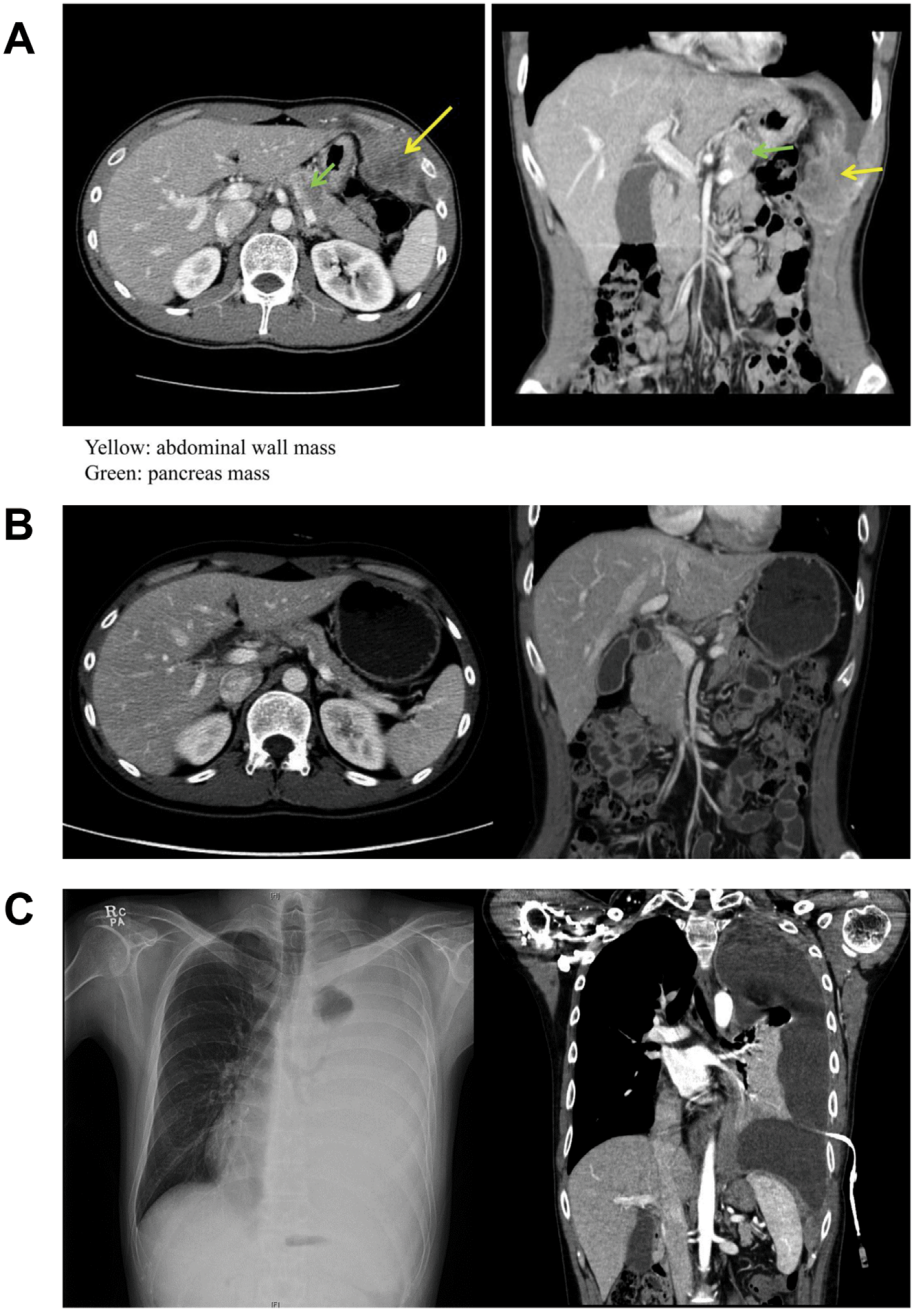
Computed tomography (CT) findings during the course of the disease. (A). Abdominal wall mass (yellow) and pancreas mass (green) at the time of initial diagnosis. (B). After 1^st^-line chemotherapy, the disease virtually disappeared. (C). A massive amount of left pleural effusion was seen after salvage chemotherapy.
